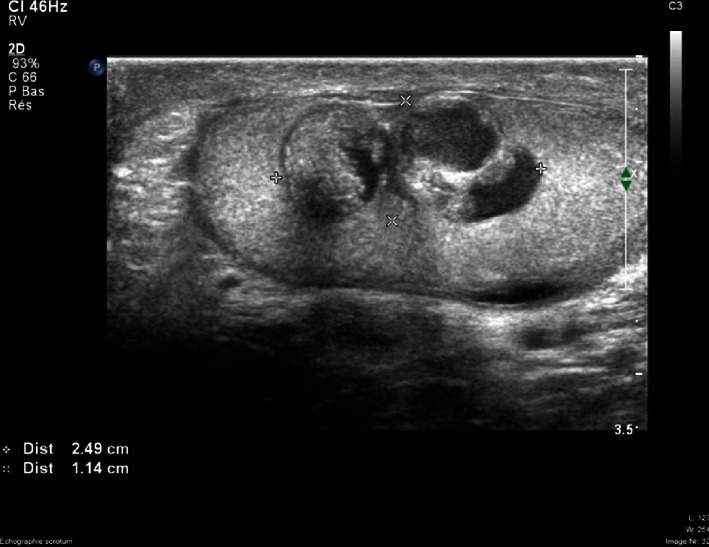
Ultrasound features: heterogenous, hypoechogenic, and cystic tumor of 20 mm.
